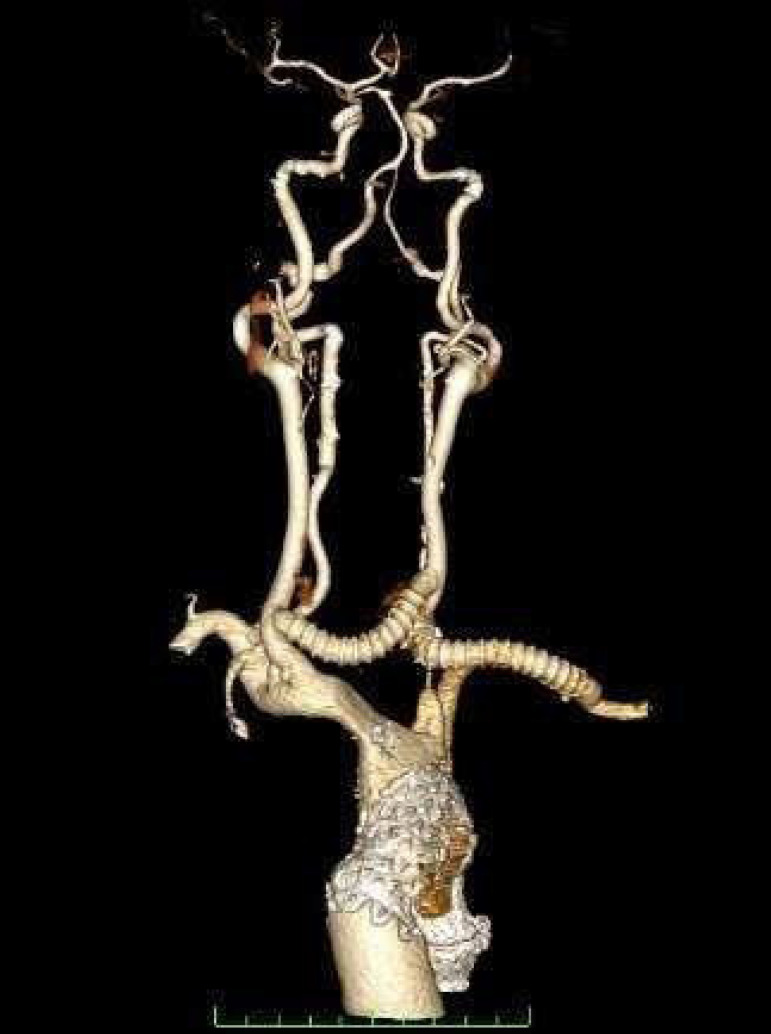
Postoperative CT angiography showing a cervical debranching with reinforced PTFE graft anastomosis from the left subclavian artery to the left carotid artery and from the left carotid artery to the right carotid artery. Image courtesy from the authors.
